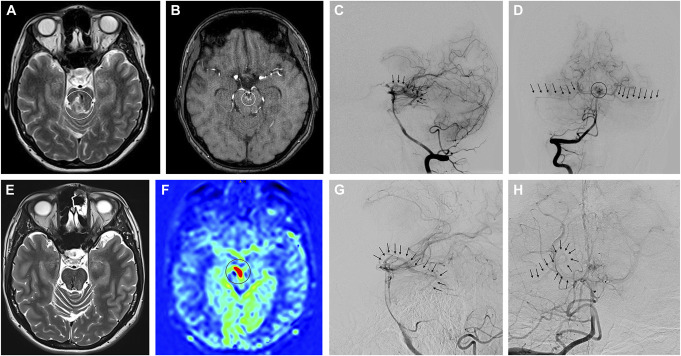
After suffering from hemorrhage (circle) of a pontine AVM (A, T2-weighted MRI, B, time of flight, circle = nidus), a patient in their 20s underwent DSA and GKRS. C (lateral view) and D (frontal view), Pre-GKRS angiograms after right vertebral artery injection (arrows = early draining veins, circle = nidus). In the standardized MRI protocol after 2 years, E, the AVM was rated obliterated according to T2 images, F, but AV-shunting (circle) was seen on arterial spin labeling. Hence, DSA was performed and the patient underwent retreatment. An early draining vein (arrows) is seen on G, lateral and H, frontal angiograms. AVM, arteriovenous malformation; DSA, digital subtraction angiography; GKRS, Gamma Knife radiosurgery.
